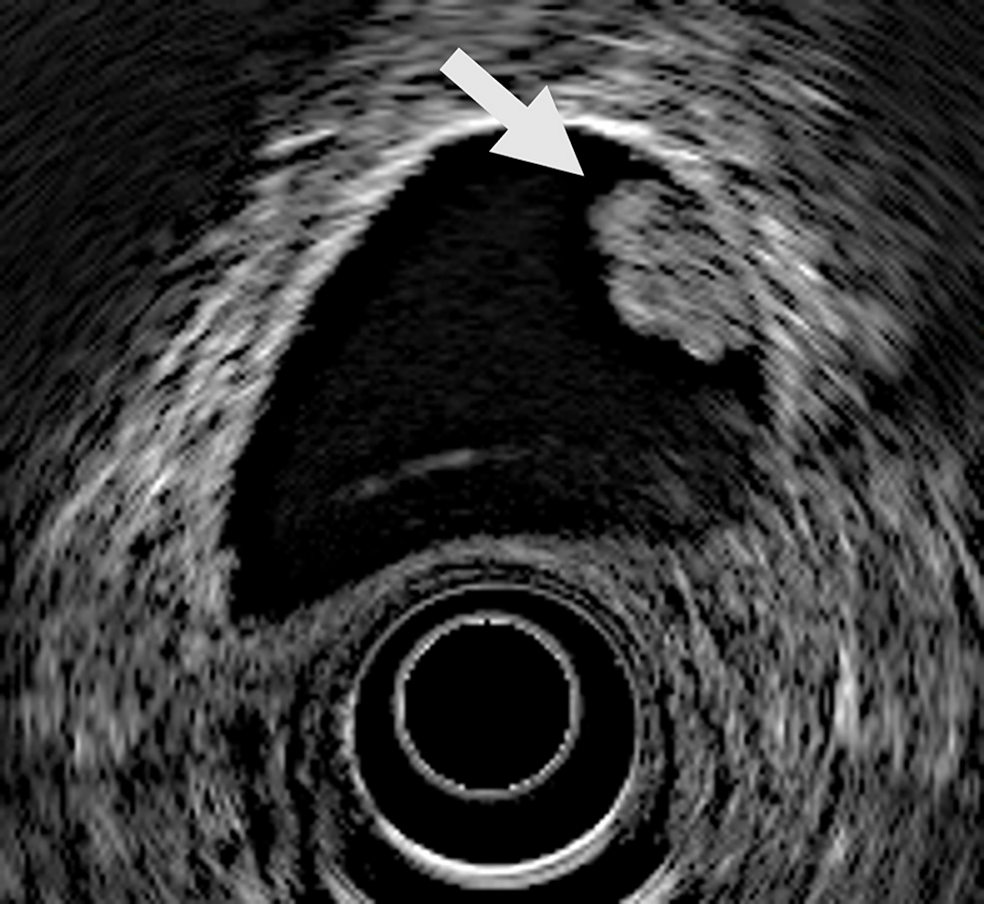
Endoscopic ultrasonography Endoscopic ultrasonography demonstrated a 10-mm brightly echogenic, pedunculated, intraluminal polypoid lesion without foci.
